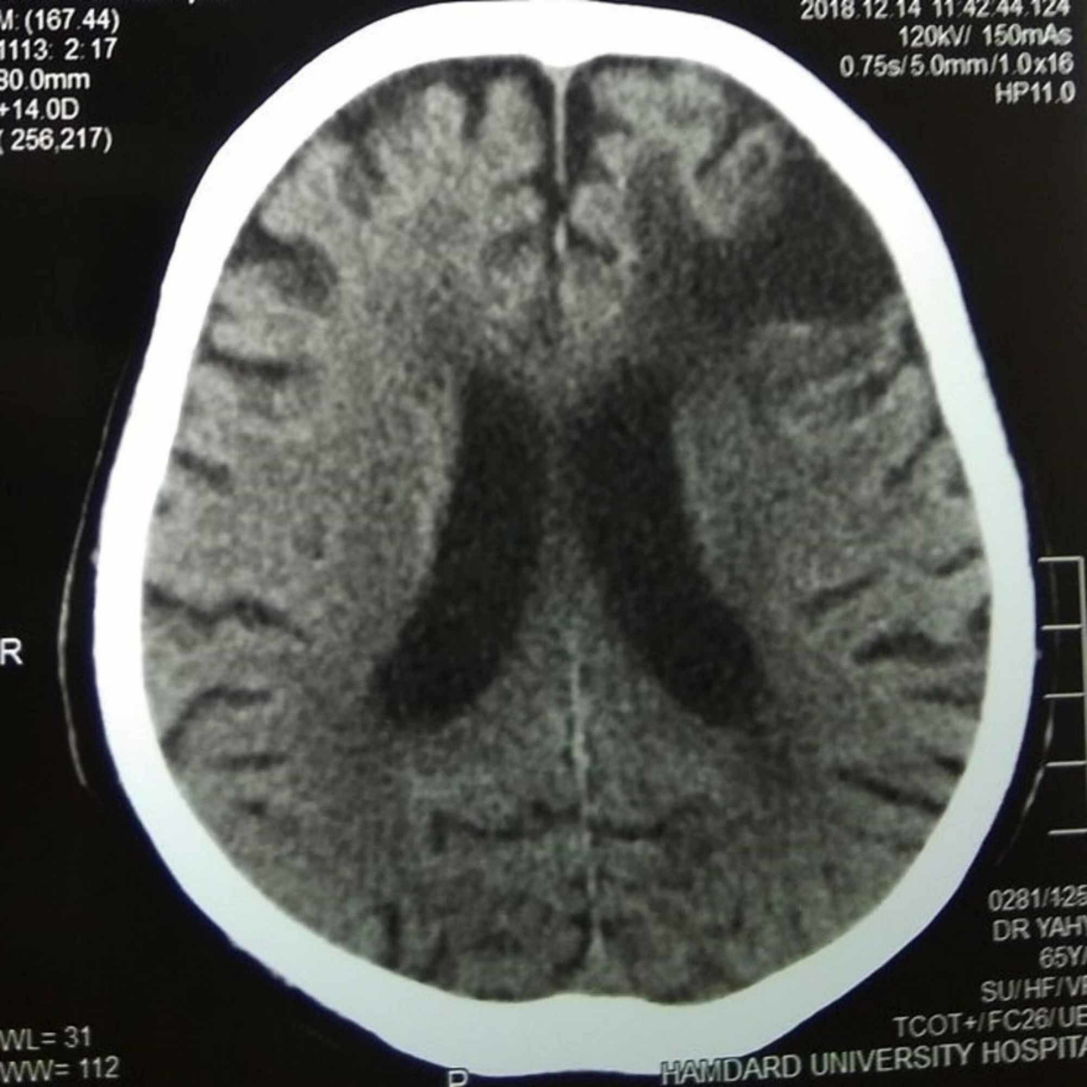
CT scan of the brain (plain, axial view), showing a left frontal infarct
